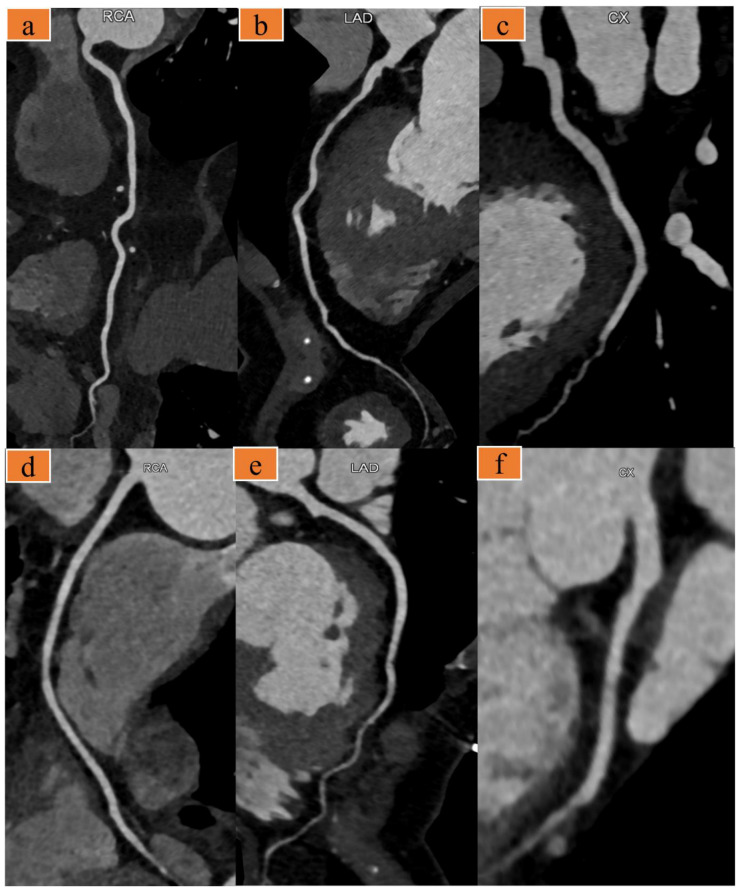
(a–c) A 91-year-old man with chief complaint of chest pain (heart rate: 67 bpm). Images were acquired with the optimized scanning scheme. Curved multiplanar reformat computed tomography (CT) images show the right coronary artery (a), left anterior descending artery (b), and left circumflex artery (c). (d–f) A 63-year-old woman presented to the emergency room with several days of chest pain, heart rate 58 bpm. Images were acquired with the traditional triple-rule-out computed tomography angiography (TRO-CTA). Curved multiplanar reformat CT images of the right coronary (d), left anterior descending artery (e), and left circumflex artery (f).
